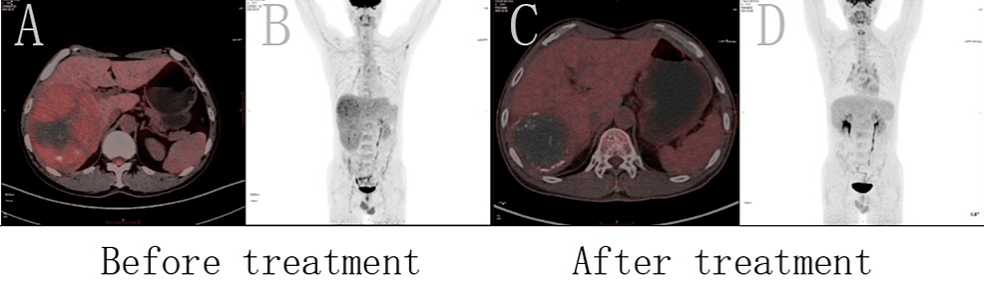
After TACE treatment with targeted immunotherapy, the patient's lesions were significantly smaller than before with decreased metabolism. A. Fusion image of PET-CT before treatment; B. MIP image before treatment; C. Fusion image of PET-CT after treatment; D. MIP image after treatment. TACE: transarterial chemoembolization; PET-CT: positron emission tomography-computed tomography scan; MIP: maximum intensity projection
